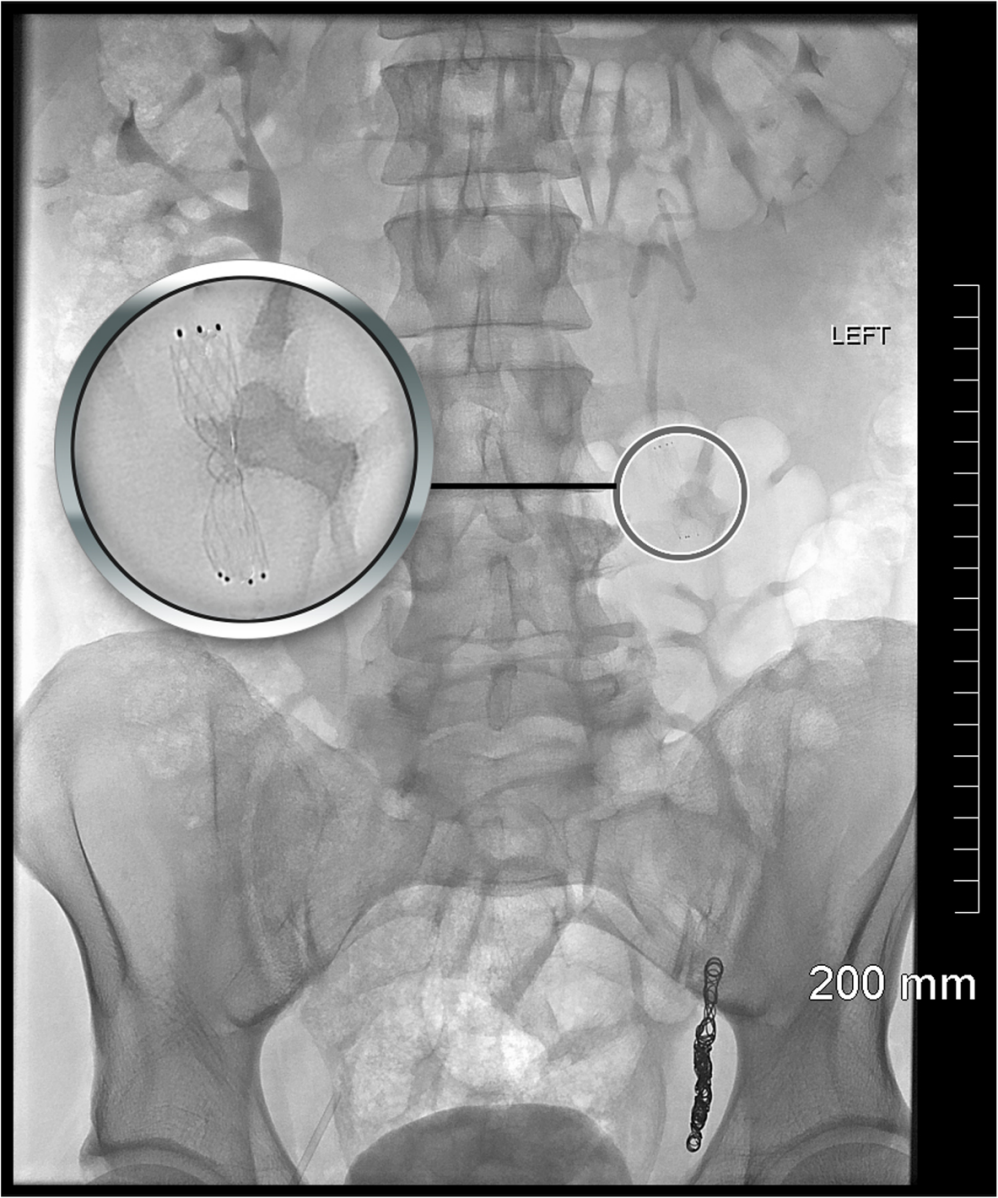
Single radiographic image with magnification over the device in situ within the left gonadal vein
